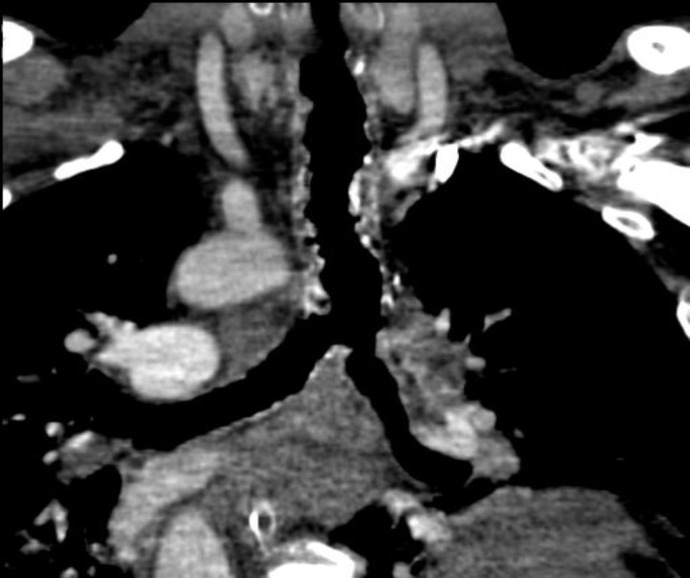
Coronal reconstructed CT scan reveals focal tracheal thickening and irregularity associated with multiple calcified nodules arising from inner wall
